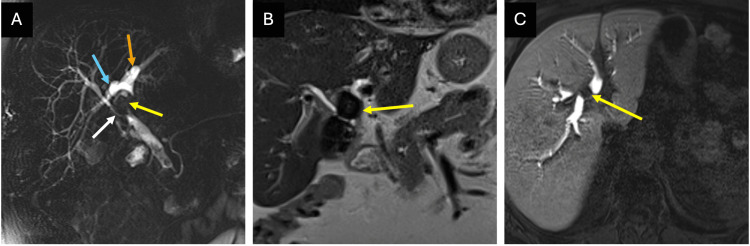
Preoperative magnetic resonance cholangiopancreatography (MRCP) findings. (A) Coronal (projectional) T2-weighted HASTE sequence demonstrates a calculus at the gallbladder neck extruding into the hepatic hilum (yellow arrow) and outlines variant anatomy: the right posterior sectoral duct (blue arrow) drains into the left hepatic duct (orange arrow), while the right anterior sectoral duct drains low into the common hepatic duct (white arrow). Recognition of this variant is important as it may influence stone distribution and increases the complexity of dissection around the confluence. (B) Coronal T2-weighted HASTE sequence demonstrates a calculus (yellow arrow) extending superior to the level of the cystic duct toward the porta hepatis, causing compression of ducts at the hepatic duct confluence. A true intraductal stone position via fistulation was only definitively confirmed at surgery. (C) Axial T1-weighted 3D VIBE sequence after gadoxetate disodium (Primovist) demonstrates a filling defect within the left hepatic duct (yellow arrow). In retrospect, this finding was consistent with intraductal stone migration through a cholecystobiliary fistula at the confluence.
